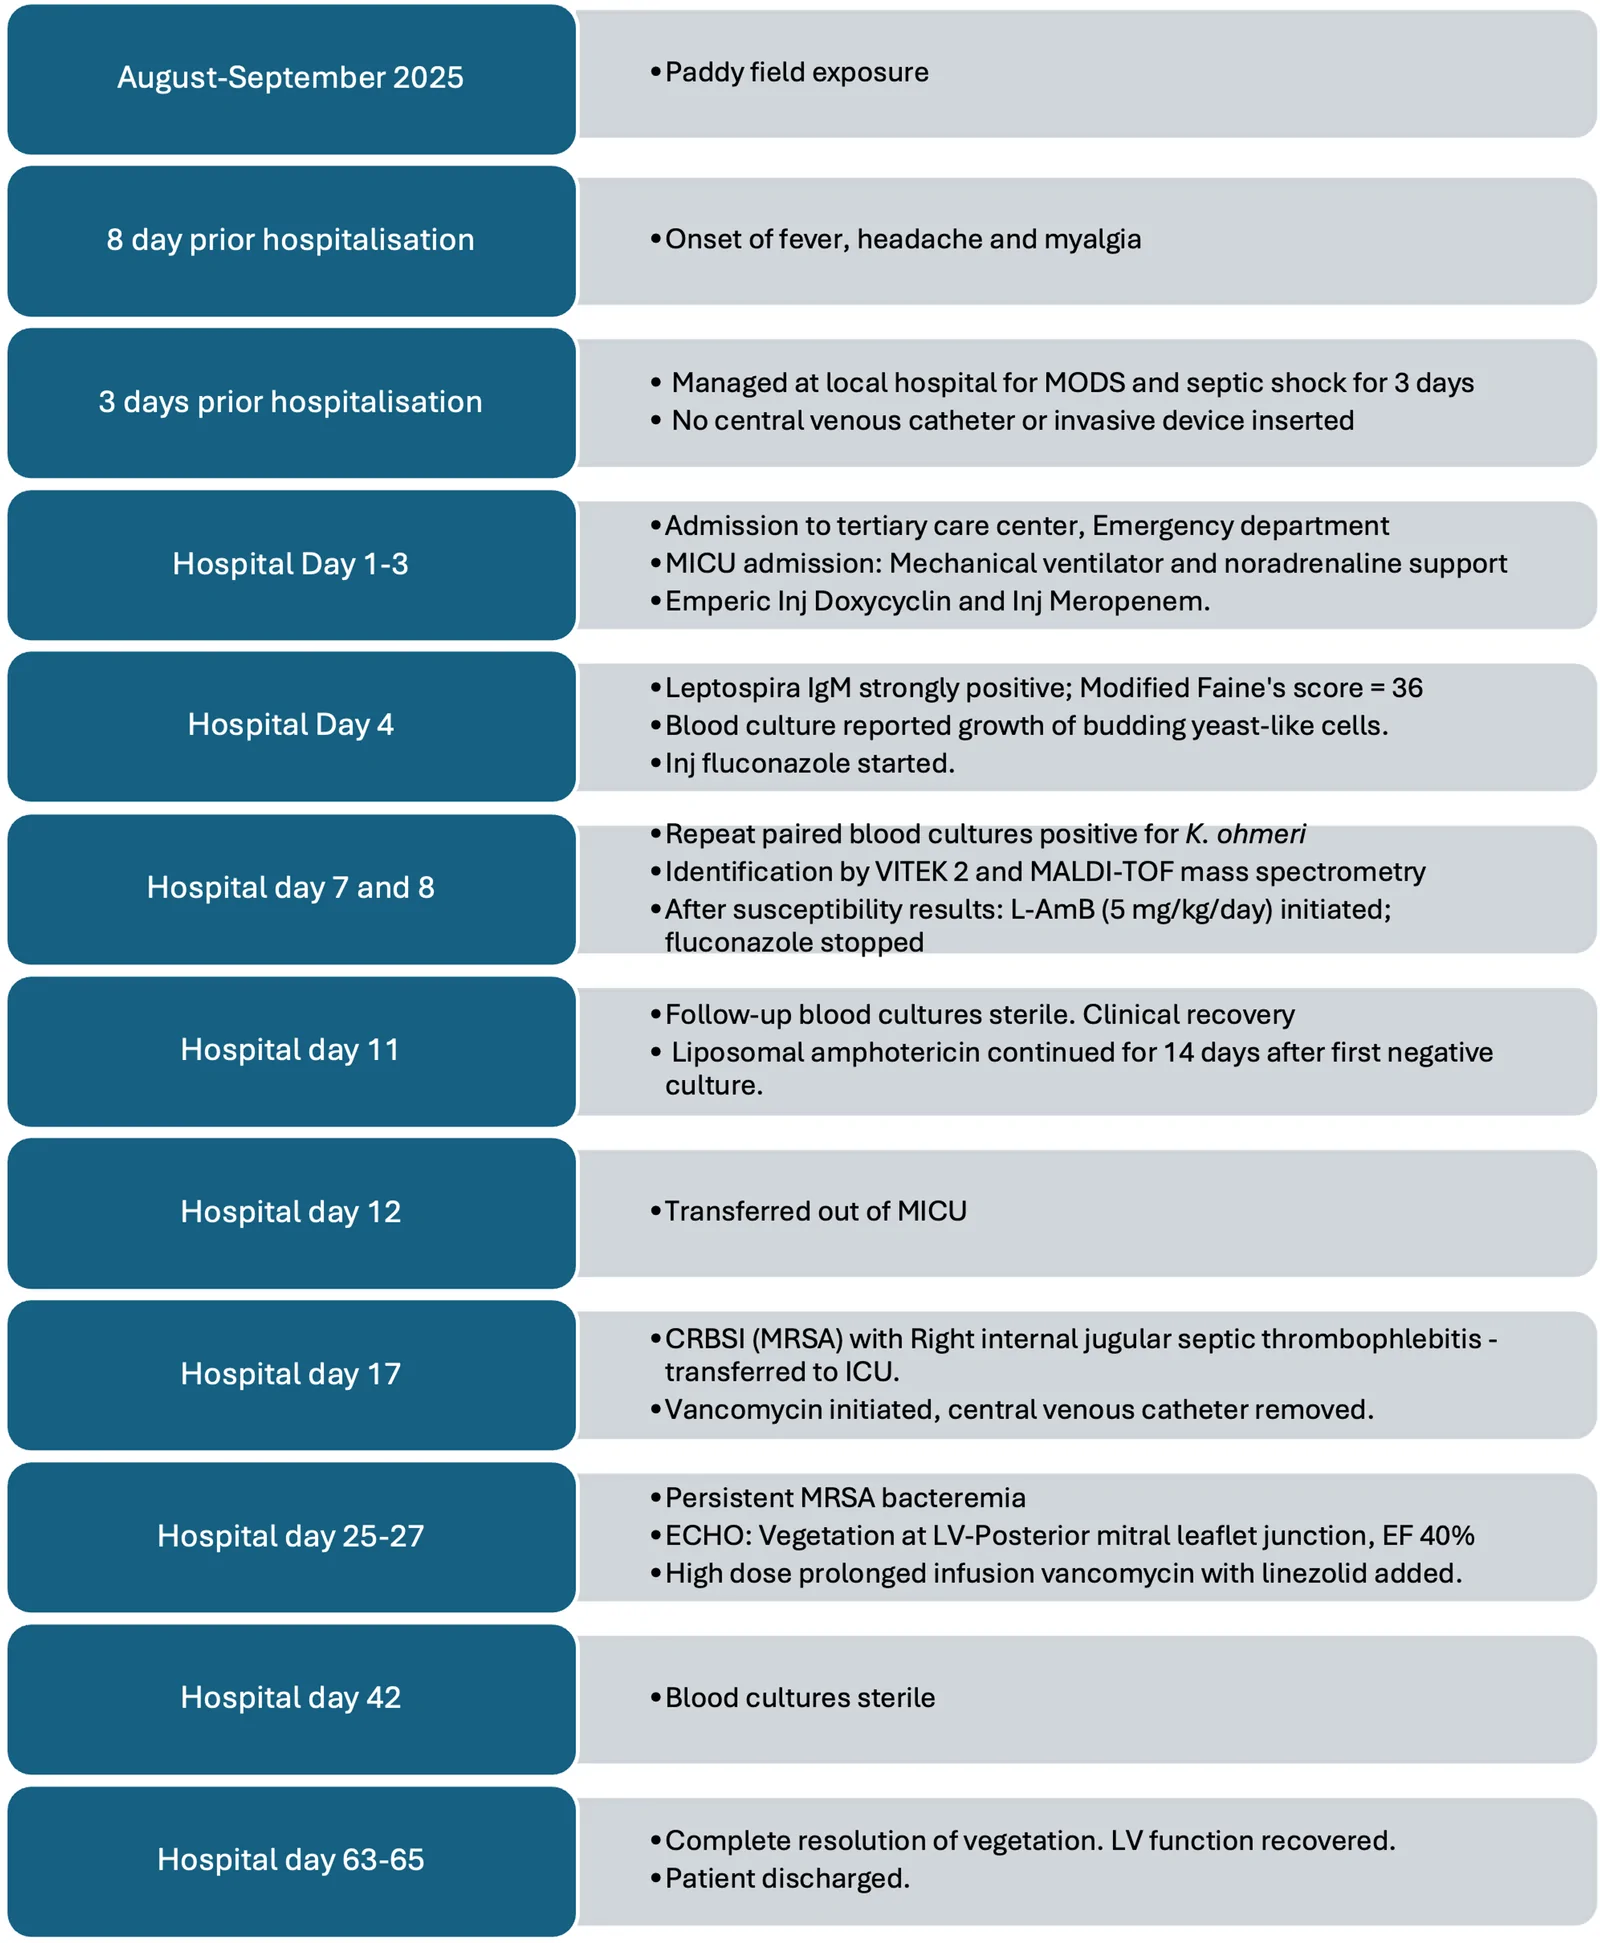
Clinical timeline of events MICU: Medical Intensive Care Unit; IgM: Immunoglobulin M; MODS: Multi-Organ Dysfunction Syndrome; L-AmB: Liposomal Amphotericin B; MRSA: Methicillin-Resistant Staphylococcus aureus; CRBSI: Catheter Related Bloodstream Infection; LV: Left Ventricle; EF: Ejection Fraction; Inj: Injection; ECHO: Echocardiogram
